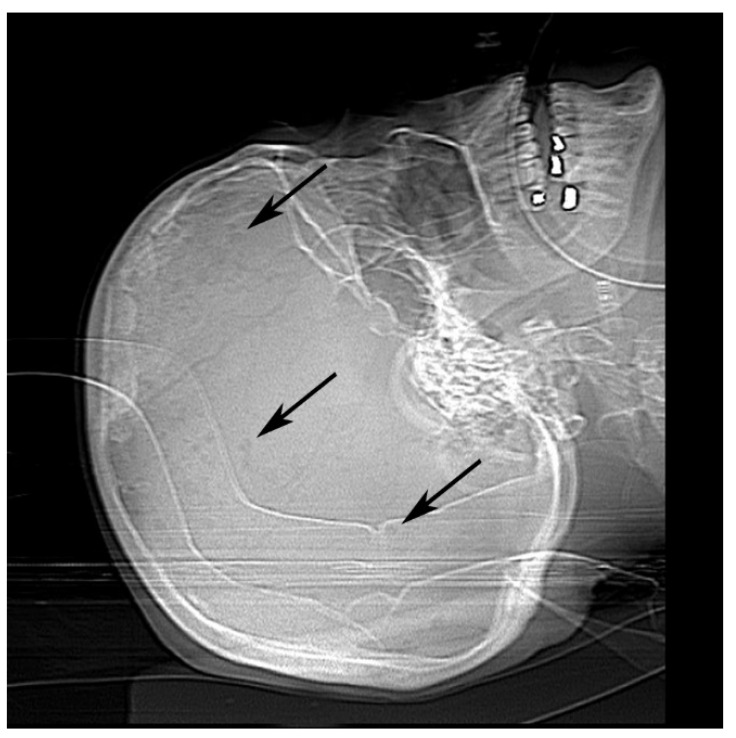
Antemortem X-ray of calvarium showed multiple punctate lucencies (black arrows).
